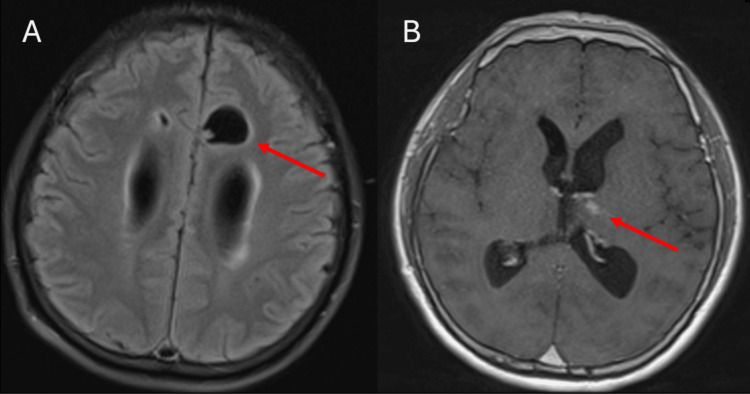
MRI of the brain on hospital day eight. A: Axial T2 fluid-attenuated inversion recovery imaging shows a 2.1 cm dominant cystic lesion with a small peripheral solid nodule, along the parasagittal left frontal lobe concerning for the active vesicular stage of the disease. B: Axial T1 post-contrast imaging shows one of several small areas of parenchymal enhancement in the left thalamus concerning for active disease.
